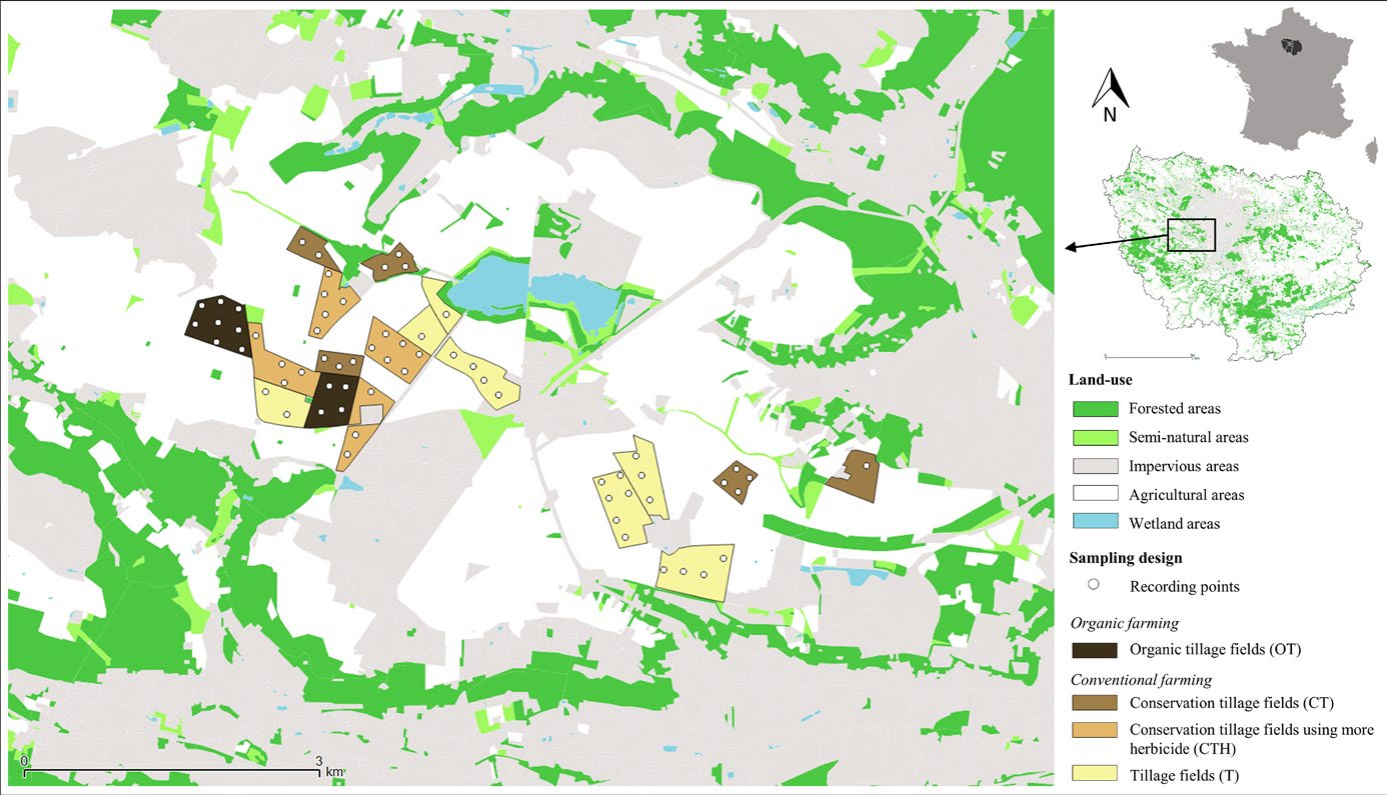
Land‐use map of the study area showing sampling sites inside wheat fields of the four studied farming systems (OT, organic tillage fields; CT, conservation tillage fields; CTH, conservation tillage fields using more herbicide; T, tillage fields)
